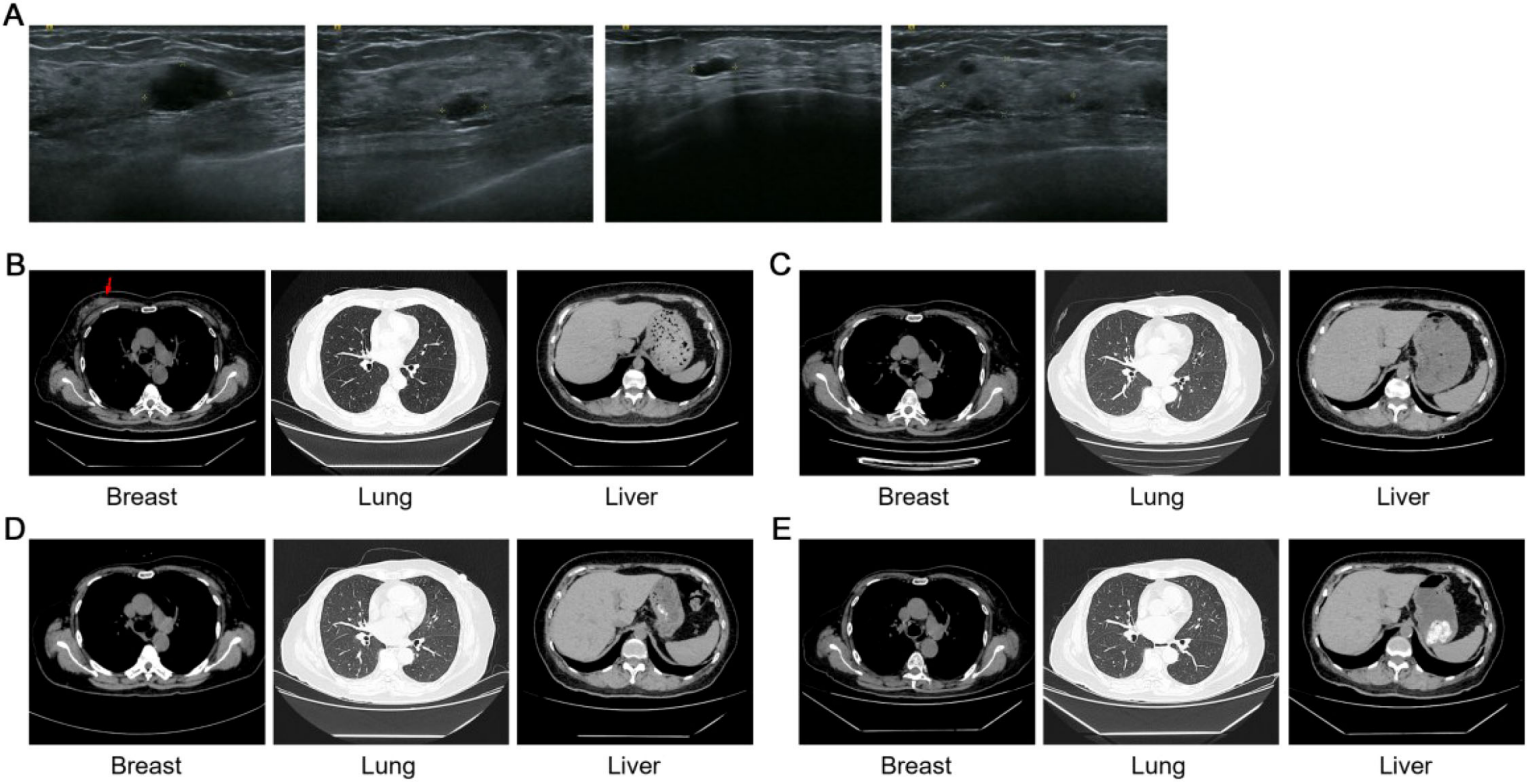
Imaging examination in the TNBC case. (A) Representative CDU images of the right breast. (B-E) Representative CT images of breast, lung and liver on June 22, 2021 (B), June 6, 2022 (C), July 20, 2023 (D) and July 19, 2024 (E), respectively.
